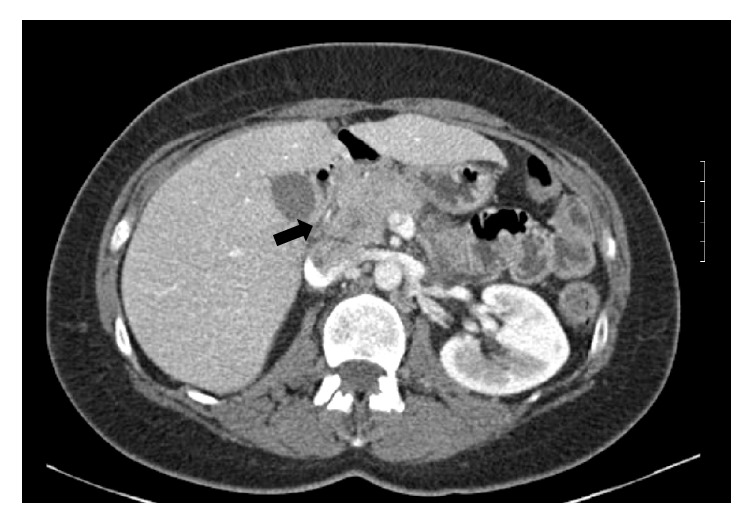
Computed tomogram of the abdomen and pelvis with contrast show abnormal fullness in the region of the pancreatic head measuring 4 × 1.5 cm (black arrow) with a dilated pancreatic duct measuring 5 mm.
